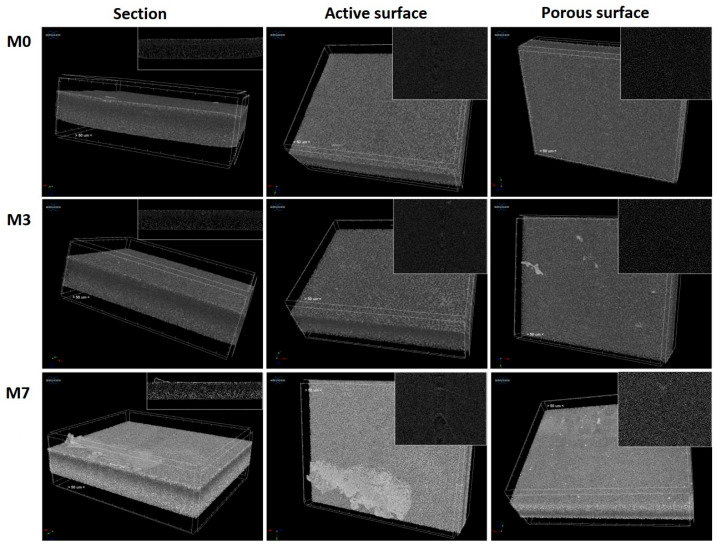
CT images obtained for the neat (M0), argon/ammonia (M3), and argon (M7) plasma-treated membranes. The scale bar represents 50 μm.
